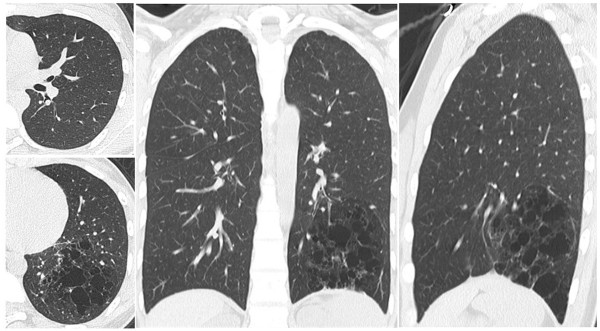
High-resolution computed tomography of the lung. Congenital pulmonary airway malformation was detected in the basal segment of left inferior lobe with a severely atretic interlobar fissure. The lesion consisted of multiple cysts with no abnormal vessels.
